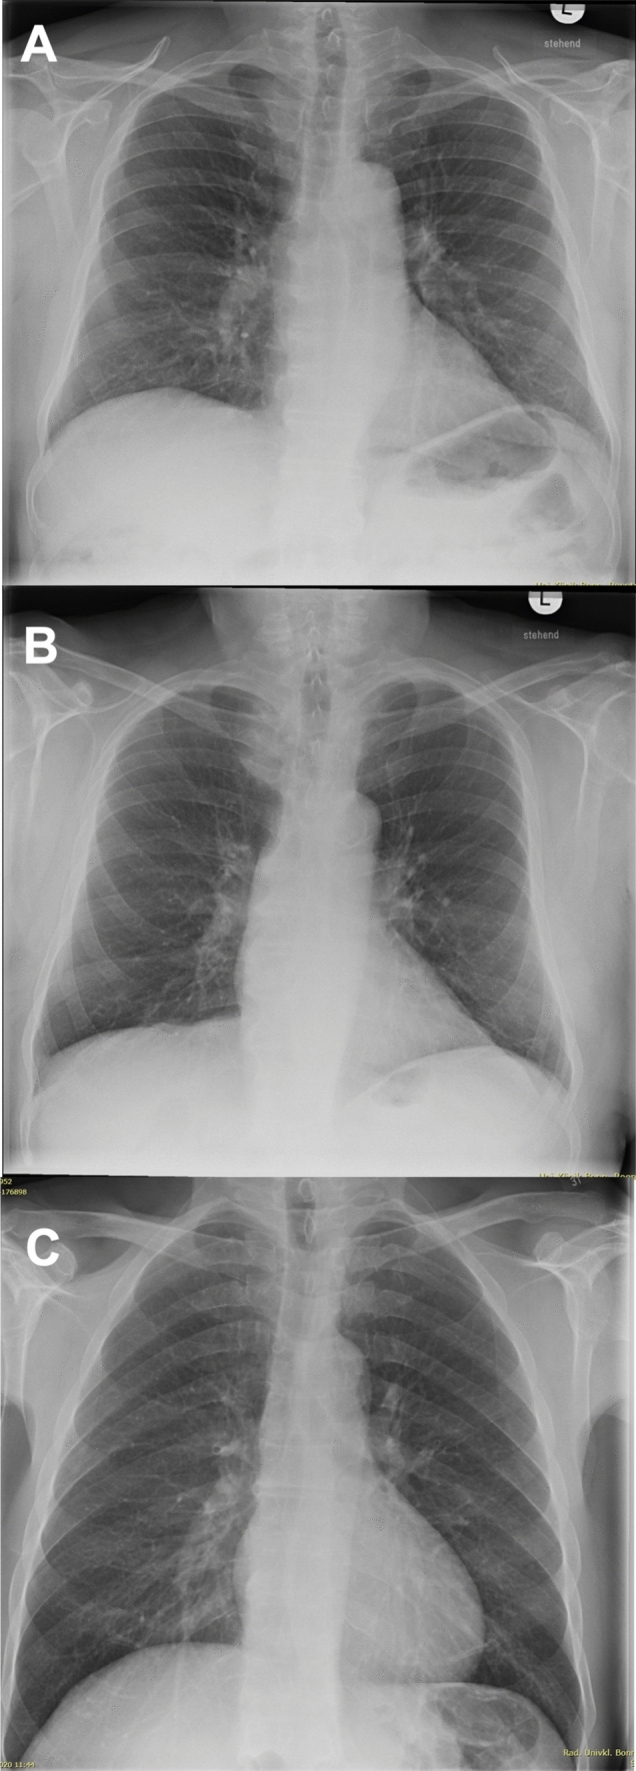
Findings in Chest Radiography. Figure 2 presents examples of typical findings in patients with arthritis in conventional posteroanterior chest radiography. A No pathological findings in a patient with rheumatoid arthritis, B Pleural scarring at the right costophrenic angle and fibrous strands in the lingular segment, interpreted as radiographic manifestations, in an asymptomatic patient with rheumatoid arthritis. C Irregular interstitial markings increase in the lung bases, interpreted as radiographic manifestations, in a symptomatic patient (cough) with rheumatoid arthritis
